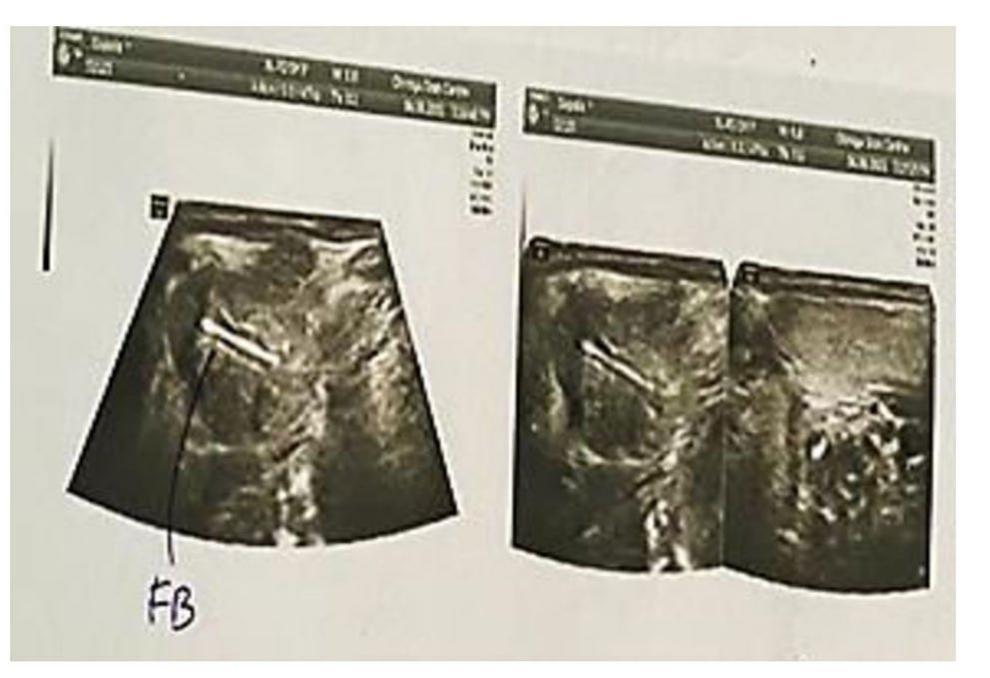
Ultrasound neck showing foreign body in right submandibular gland
